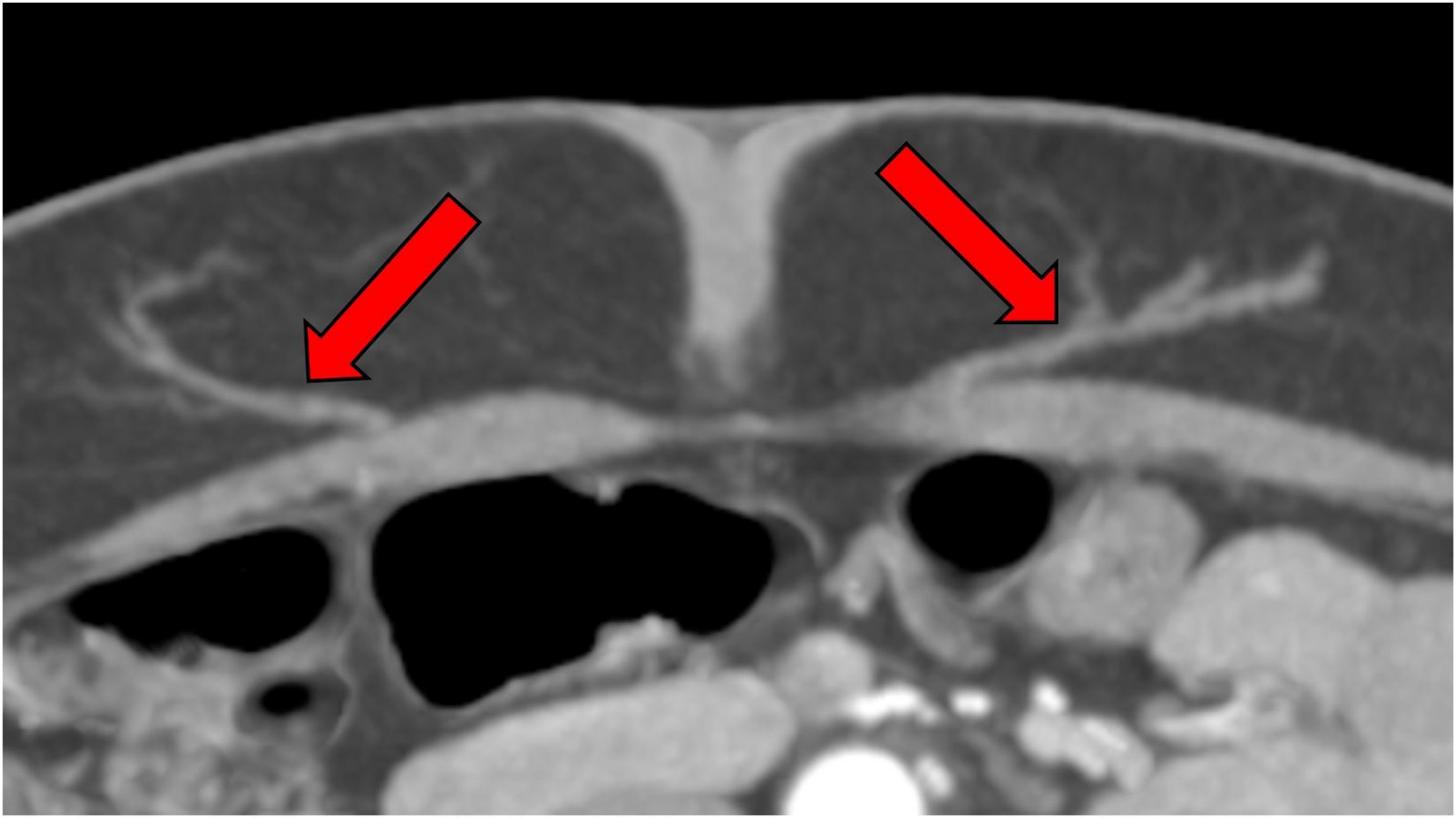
CTA-based perforator mapping enabling precise identification of vessel course and caliber, which facilitates tailored flap design and minimizes intraoperative dissection time.
